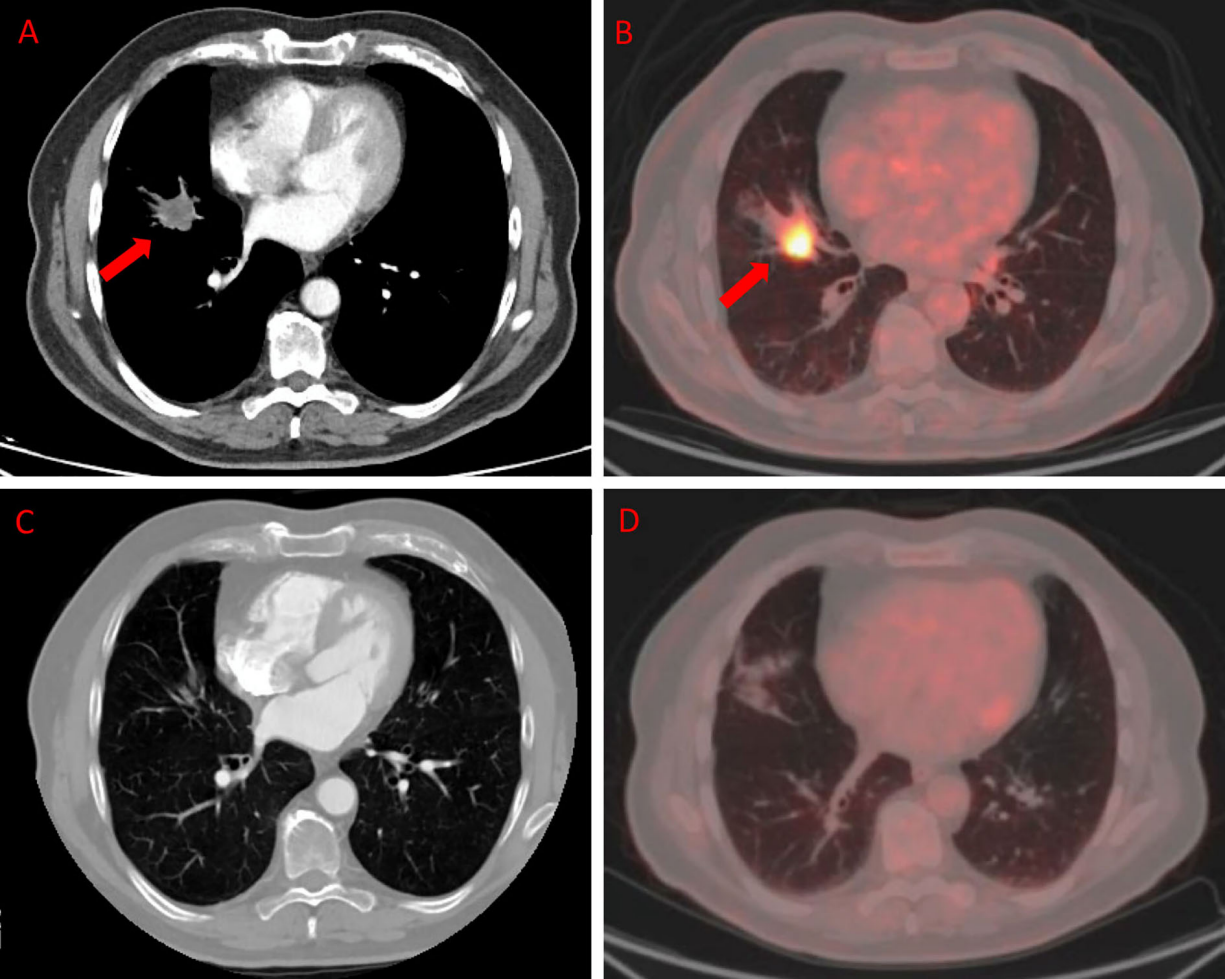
ceCT and ^18^F-FDG PET/CT in a patient affected by colon cancer with lung metastasis. A 79-year-old male affected by colorectal cancer with lung metastasis, subjected to 12 cycles of Bevacizumab-based chemotherapy and 24 DEHY sessions on the thorax as first-line therapy. Baseline ceCT (A) showed metastasis in the middle lobe (red arrows). Baseline whole body ^18^F-FDG PET/CT (B) confirmed lung involvement by the increased ^18^F-FDG uptake (red arrows) detectable axial fused PET/CT images in the same site. Timepoint-2 ceCT (C) and ^18^F-FDG PET/CT (D) evaluation demonstrated CR of lung metastasis.
